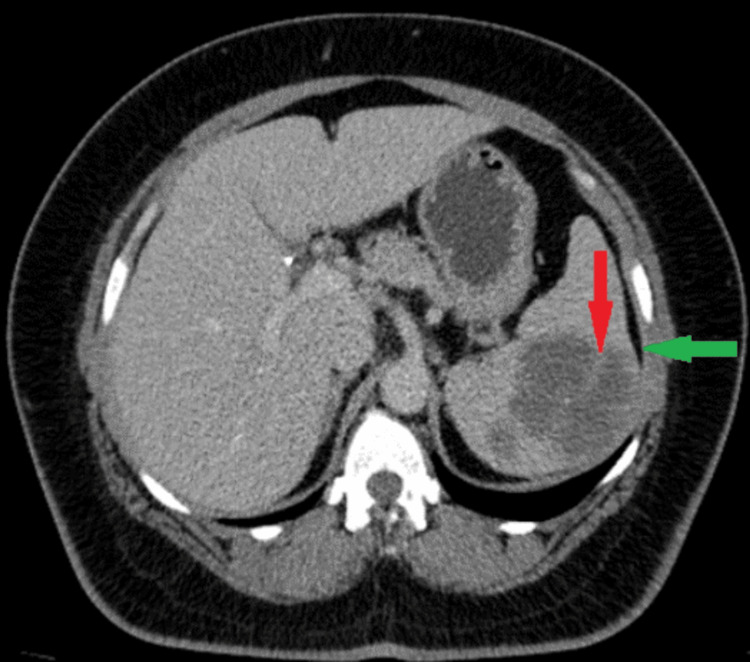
CT abdomen with IV contrast-portal phase, in axial plane, showed splenic, subcapsular, heterogenous predominantly hypodense lesion with internal streak enhancing components (red arrow), irregular contour of the lesion, and mild bulging of capsule was noted (green arrow).
